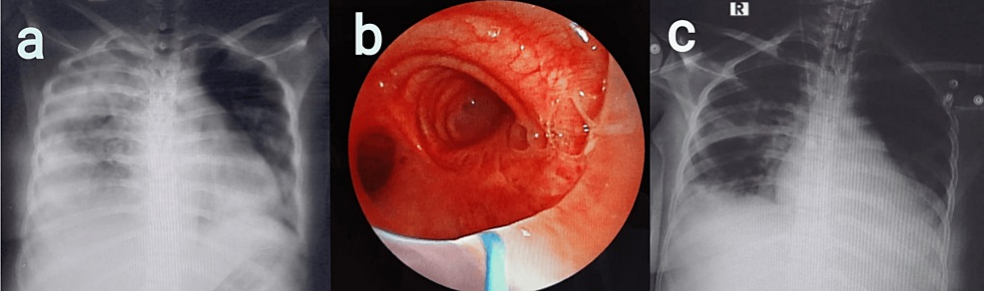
a) Chest radiograph revealed white-out lung on the right side, b) bronchoscopy revealed large mucus plugs obscuring the right mainstem bronchus and right upper lobe (RUL) bronchus, and c) post-bronchoscopy chest radiograph revealed lung expansion with a resolution of the collapse
